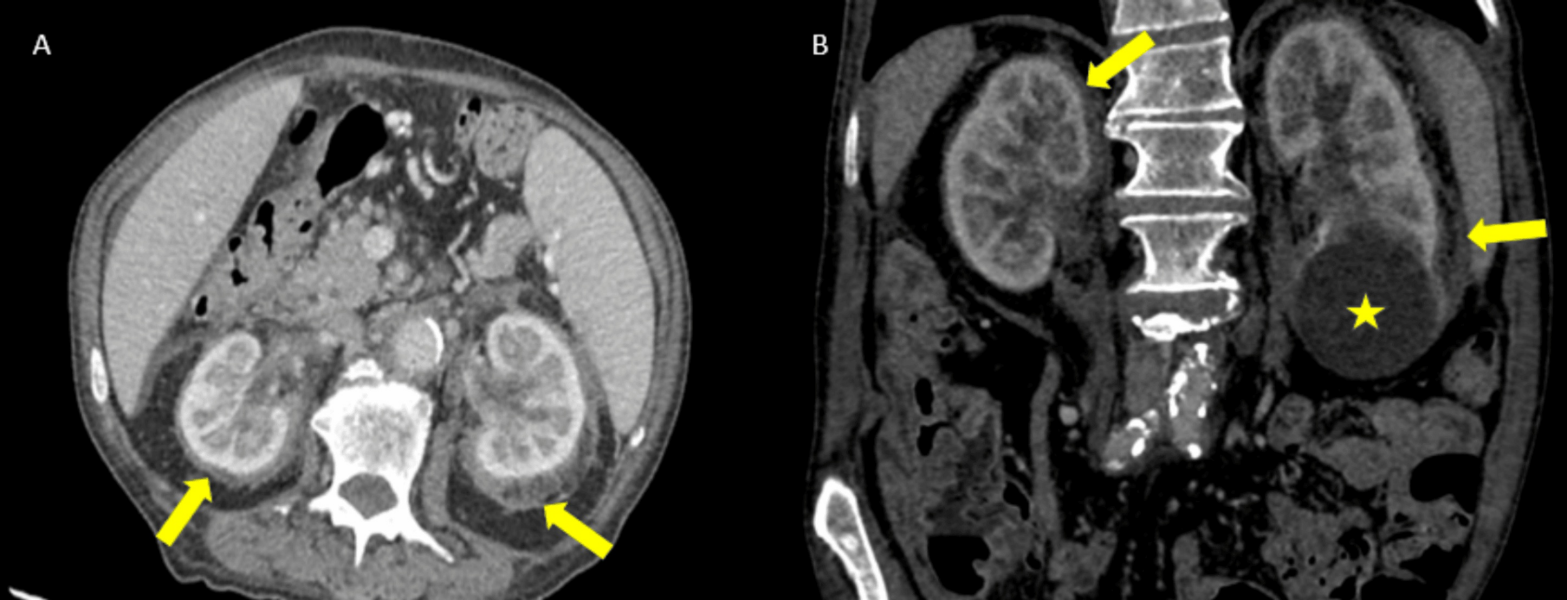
Axial CT (A) and coronal reformation depicts bilateral infiltration of the perirenal fascia – hairy kidney sign (yellow arrows). Large simple cortical cyst is also seen in the lower pole of the left kidney (yellow star) CT: computed tomography
